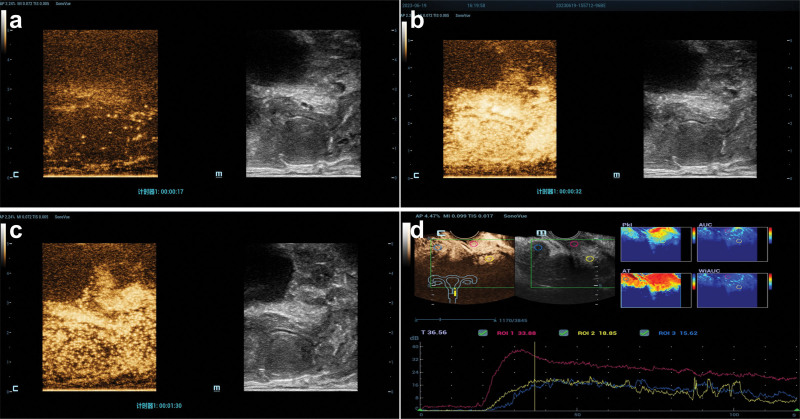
Transvenous contrast-enhanced ultrasound images. (A-C) After contrast agent injection, the mass began to show “centripetal” filling at approximately the 17th second, reached the peak at approximately 32 s, and exhibited slow, regular fading in the late phase with a capsular appearance; (D) perfusion data at the mass: time to peak: 32.4 s, peak intensity: 37.6 dB, AUC: 2654 dB; perfusion data at the cervix: time to peak: 54.4 s, peak intensity: 19.4 dB, AUC: 1267 dB; perfusion data of the uterine myometrium: time to peak: 55.3 s, peak intensity: 19.0 dB, AUC: 1294 dB.
